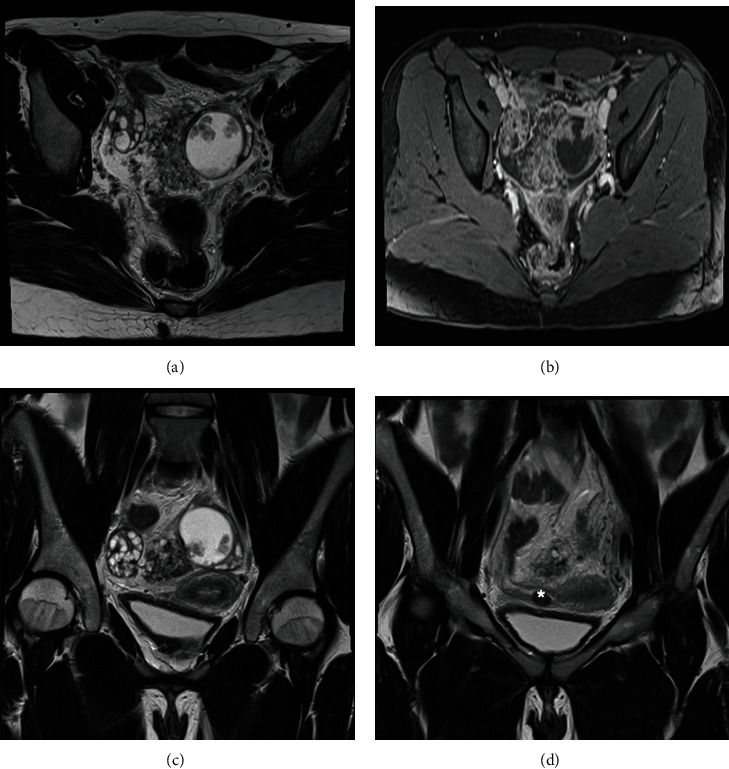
Pelvic MRI. T2-weighted axial image (a). Dynamic study (b). T2-weighted coronal image (c, d). There is a complex cystic lesion of the left ovary, with some solid papillary projections, which avidly enhance after contrast. Involving both ovaries there is another mixed mass, with the same characteristics of the previously described but arising from the right ovary. Both ovaries have multiple follicles, the right with polycystic appearance. Pedunculated leiomyoma (^∗^) of the right broad ligament. Small volume of peritoneal effusion in pelvic situation (1.5 T MRI machine).
